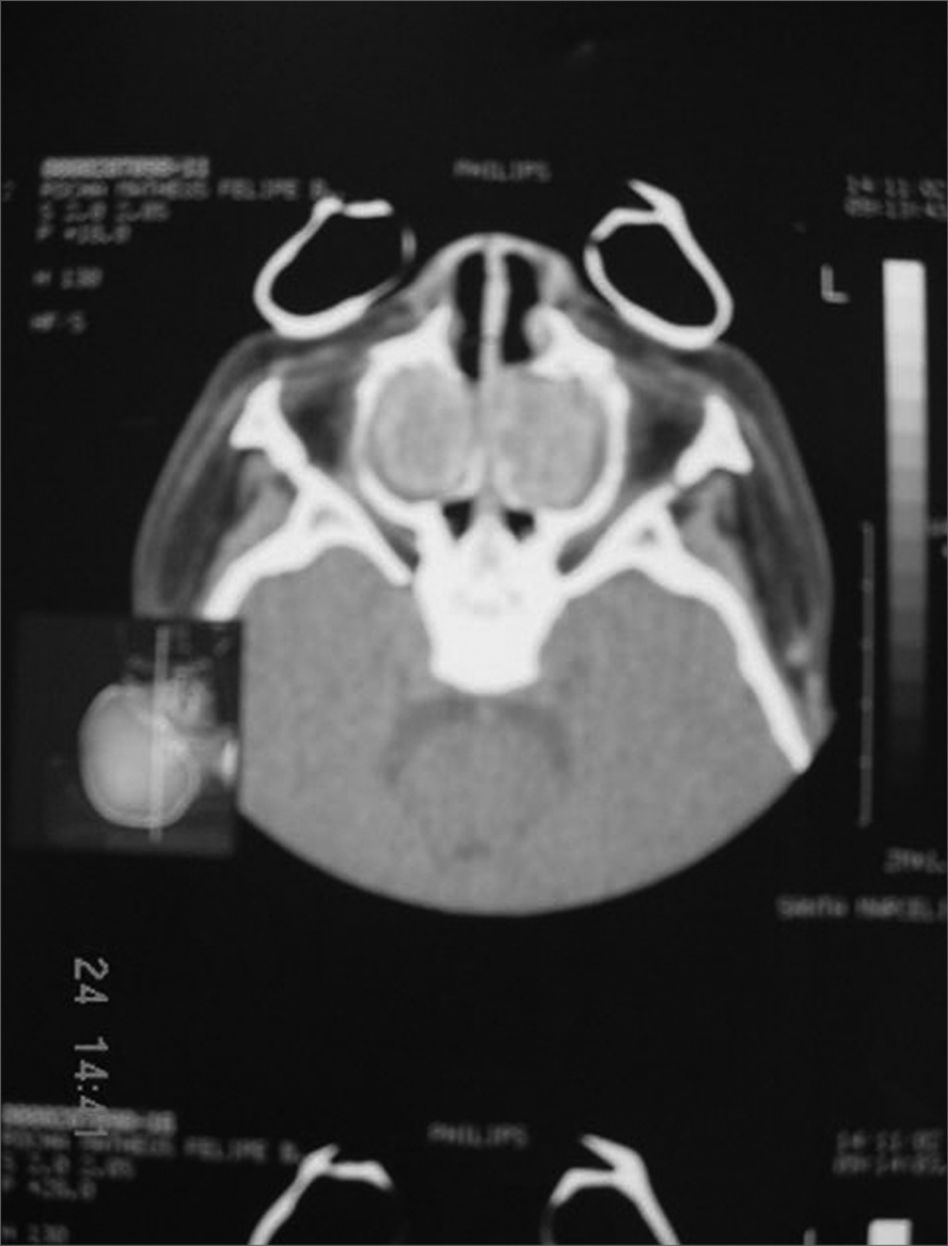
Paranasal sinuses CT Scan, axial view, soft tissue window, showing a cystic-type lesion with a peripheral halo around the maxillary sinuses, emphasizing a chronic inflammatory process. Such cystic formation causes the bulging of the lateral nasal wall and nasal cavity narrowing.
